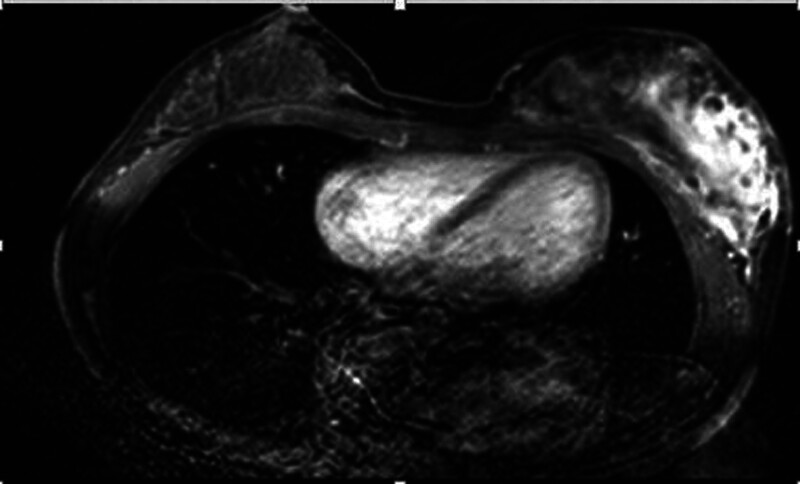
Enhanced MRI revealed pronounced heterogeneous nodular enhancement in the left breast, accompanied by infiltration of the left chest wall and thickening of the adjacent cutaneous tissue.
